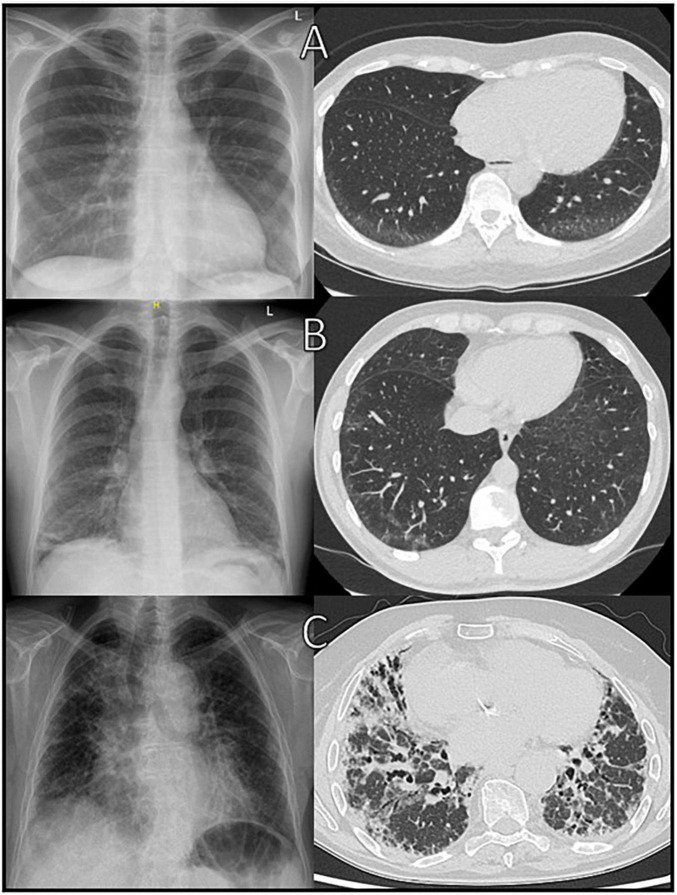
Comparison of chest X-ray with HRCT at initial diagnosis of ILD. (A) Early Sharp syndrome with ground-glass opacities in HRCT and inconspicuous chest X-ray, (B) early systemic sclerosis with ground-glass opacities, reticulations (early NSIP-pattern), and small consolidations in chest X-ray and (C) end-stage Sjögren’s syndrome with distinct consolidations in chest X-ray and beginning UIP-pattern in HRCT.
